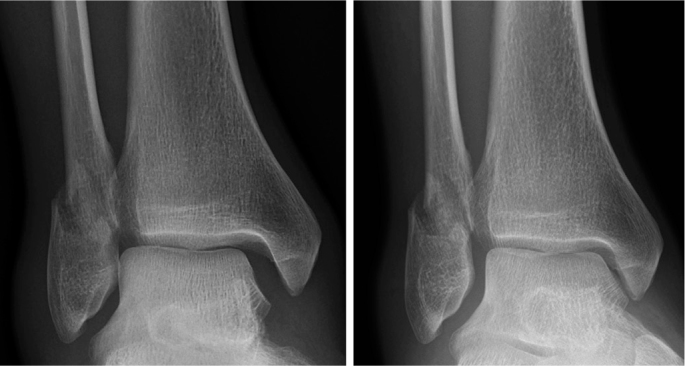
Comparison of non-weightbearing and weightbearing radiographs in a lateral malleolar fracture. Left: Widening of the medial clear space (MCS) on non-weightbearing views. Right: Restoration of ankle congruency with weightbearing.
